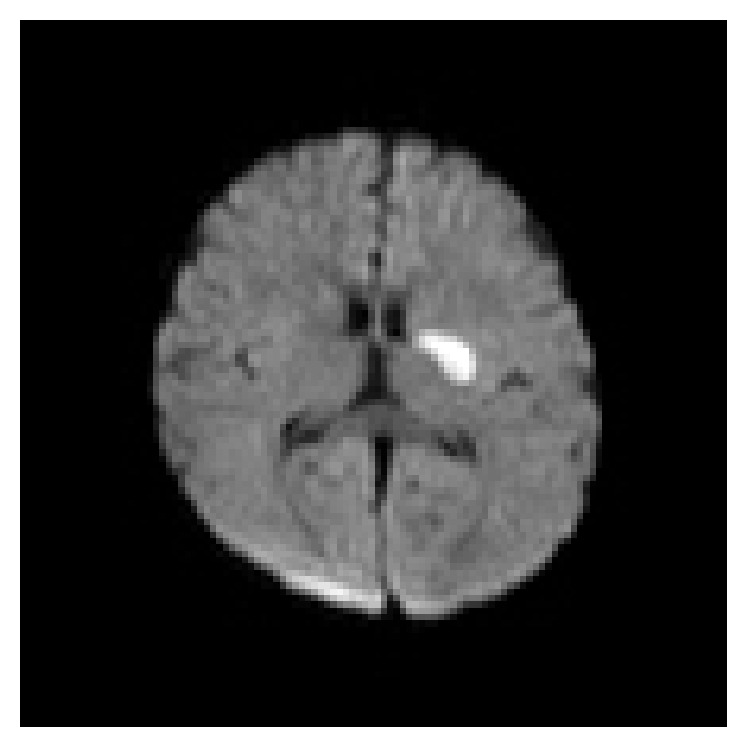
Restricted diffusion on DWI noted in left capsuloganglionic region suggestive of acute infarct.
